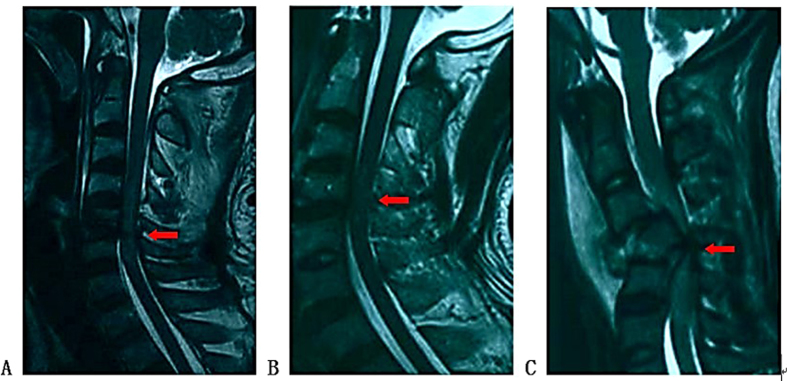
Qualitative assessment of sagittal T2 MRI. (A) Cord hemorrhage with cord swelling just below the compressed area; (B) Cord contusion; (C) Cord transection.
